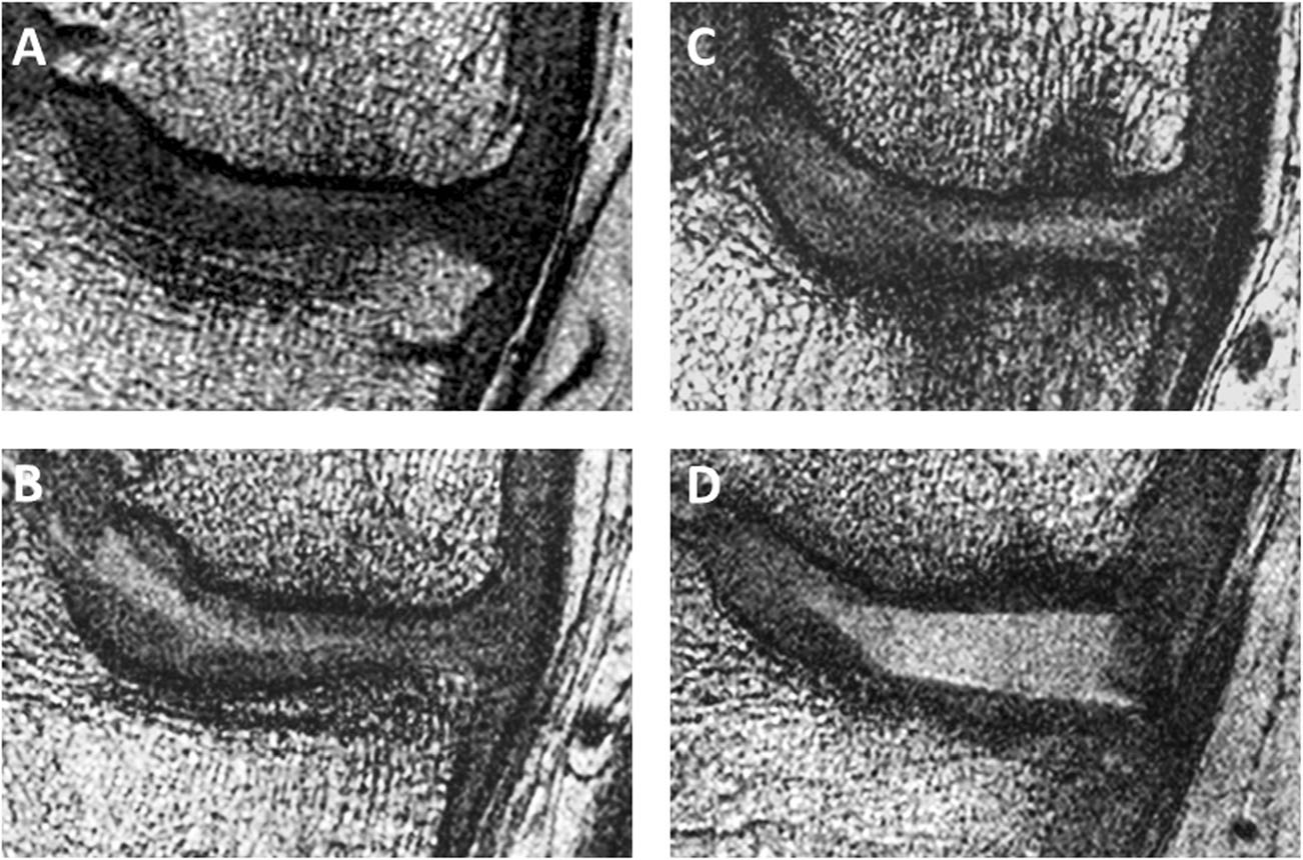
Example coronal 3D FISP MR images through the medial tibiofemoral compartment of radiographic progressors and non-progressors at the initial timepoint. *A*, non-progressor, texture score (TS) –1.22, *B*, non-progressor, TS –1.44, *C*, progressor, TS +1.01, and *D*, progressor, TS +0.93. Higher texture scores correspond to less spatially organised subchondral bone
